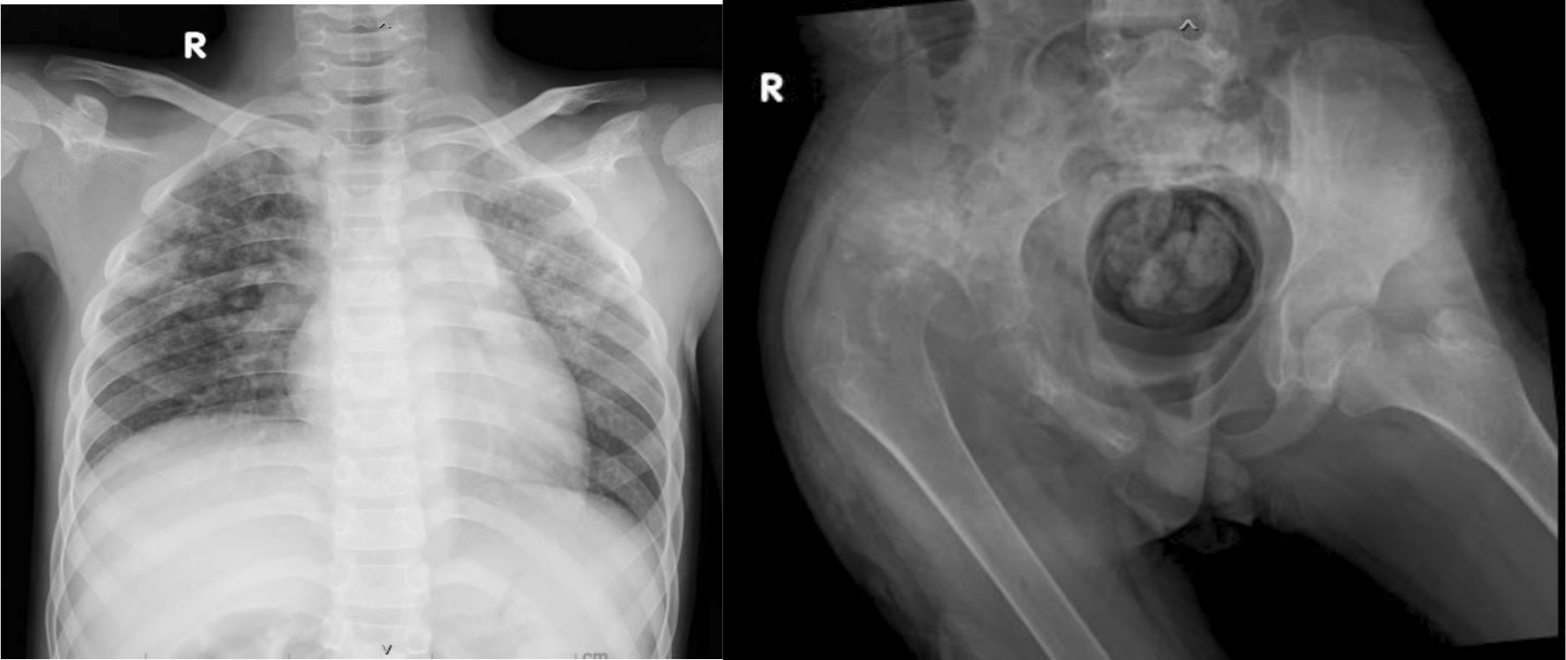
Chest and pelvic radiographs. Chest x‐ray showing bilateral patchy infiltrates in the supraparahilar regions, suggestive of pulmonary tuberculosis (Left). Pelvic radiograph showing extensive destruction of the right femoral head, neck, and acetabular roof, with posterior‐superior dislocation of the femoral head and associated soft tissue swelling, consistent with advanced tuberculous coxitis (Right).
